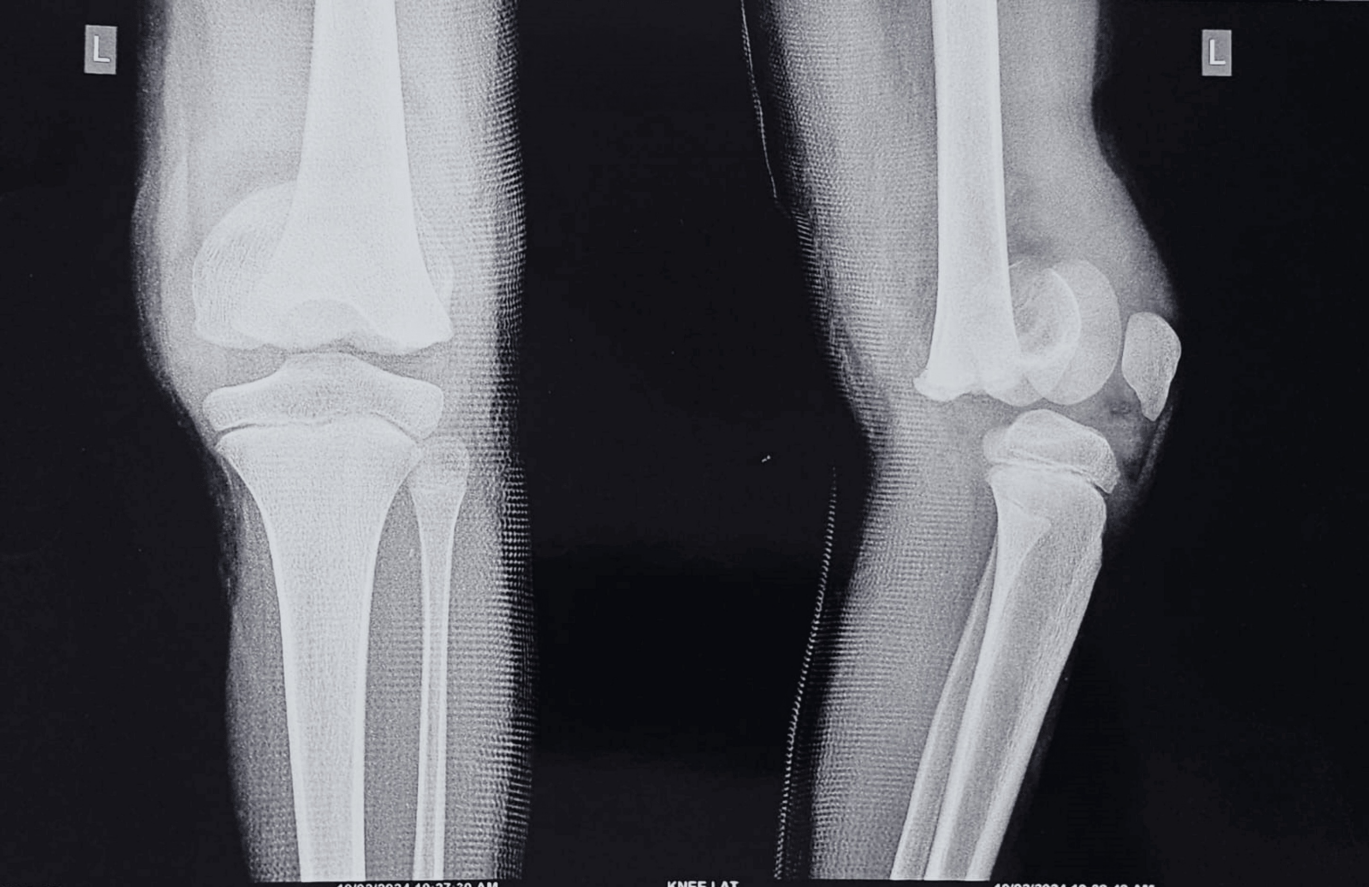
Pre-operative X-ray showing the left distal femur transphyseal fracture.
